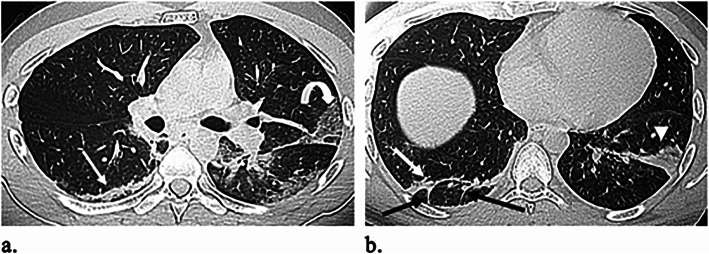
A 31-year-old male patient presented with a sore throat and dry cough. O_2_ saturation was 97%. a, b Axial unenhanced HRCT images reveal subpleural patches of consolidation (white arrowhead), crazy paving (curved arrow), subpleural lines (white arrows), and parenchyma bands (black arrows)
